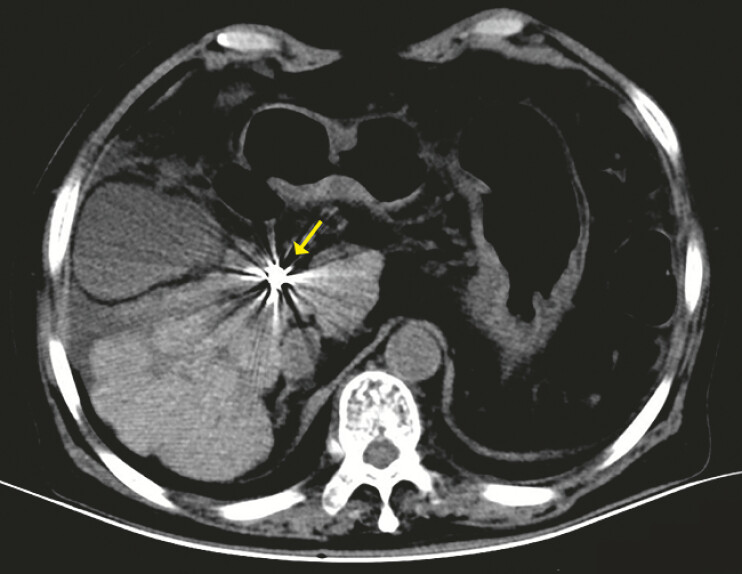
Immediate CT confirmed the coil position (yellow arrow). CT, computed tomography.
